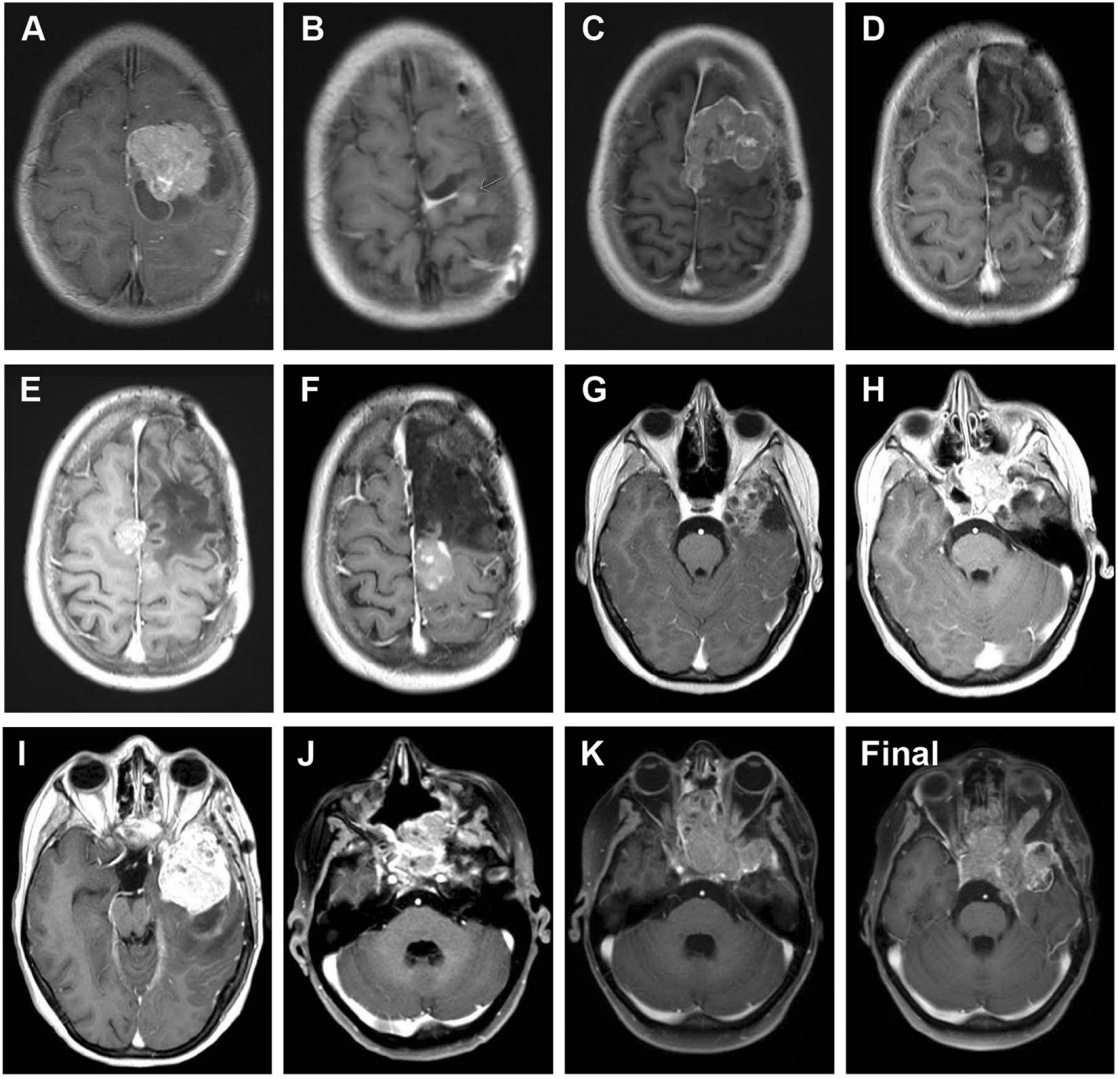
Post-contrast, T1 weighted axial MRI images of all recurrences. (A–K) representative images prior to 1^st^ to 11^th^ surgical resections respectively. (L), representative image of final tumour progression. (A) age 6.79, underwent surgical resection of left frontal tumour. (B) age 7.33, left frontal craniotomy, partial resection, treated with radiotherapy and temozolomide. (C) age 9.11, left frontal subtotal resection, treated with CCNU and temozolomide. (D) age 11.26, biopsy. (E) age 13.10, resection. (F) age 14.04, resection. (G) age 14.29, right temporal craniotomy, gross total resection. (H) age 14.67, sphenoid tumour, endoscopic endonasal sphenoidectomy. (I) age 14.96, left temporal resection. (J) age 16.05, nasal biopsy. (K) age 16.17, nasal cavity resection. The patient died aged 16.42 years (Final).
